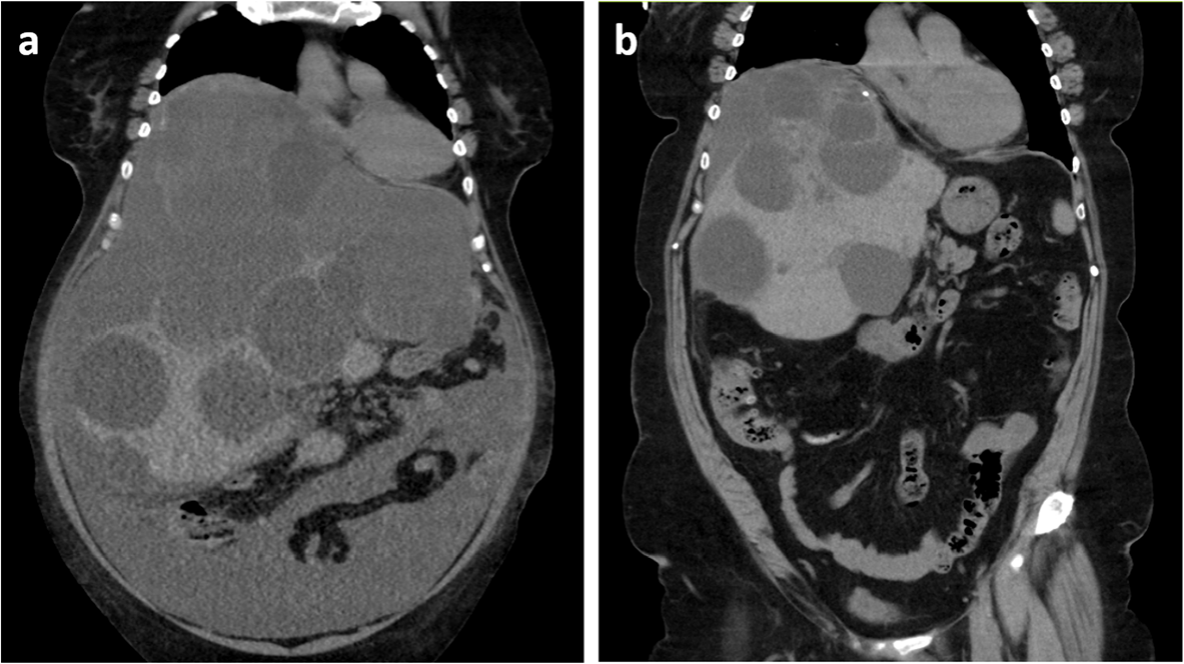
Computed tomography images (frontal section). a At the first visit of the patient, giant polycystic liver, ascites, and elevated right diaphragm were observed. b Ten months after the surgery, the volume of liver cysts was greatly reduced and ascites were not observed
